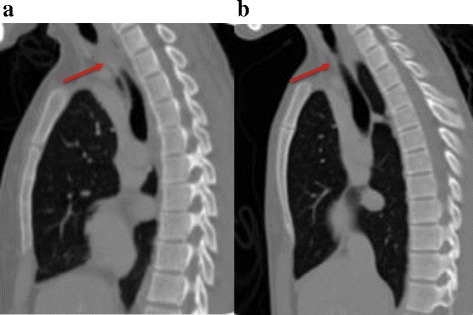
CT Thorax pre- and post-intervention. a Lateral view of a CT Thorax showing 1 cm of total stenosis at the upper one third of trachea. b Lateral view CT Thorax at 36 weeks following successful intervention
